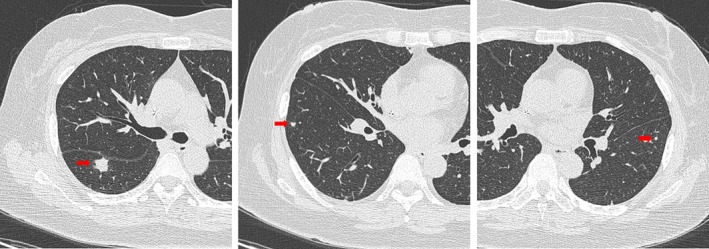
Chest computed tomography (CT) at admission showed numerous small bilateral pulmonary nodules up to 10 mm in diameter, without central necrosis, cavitation, or enlargement of mediastinal or hilar lymph nodes.
